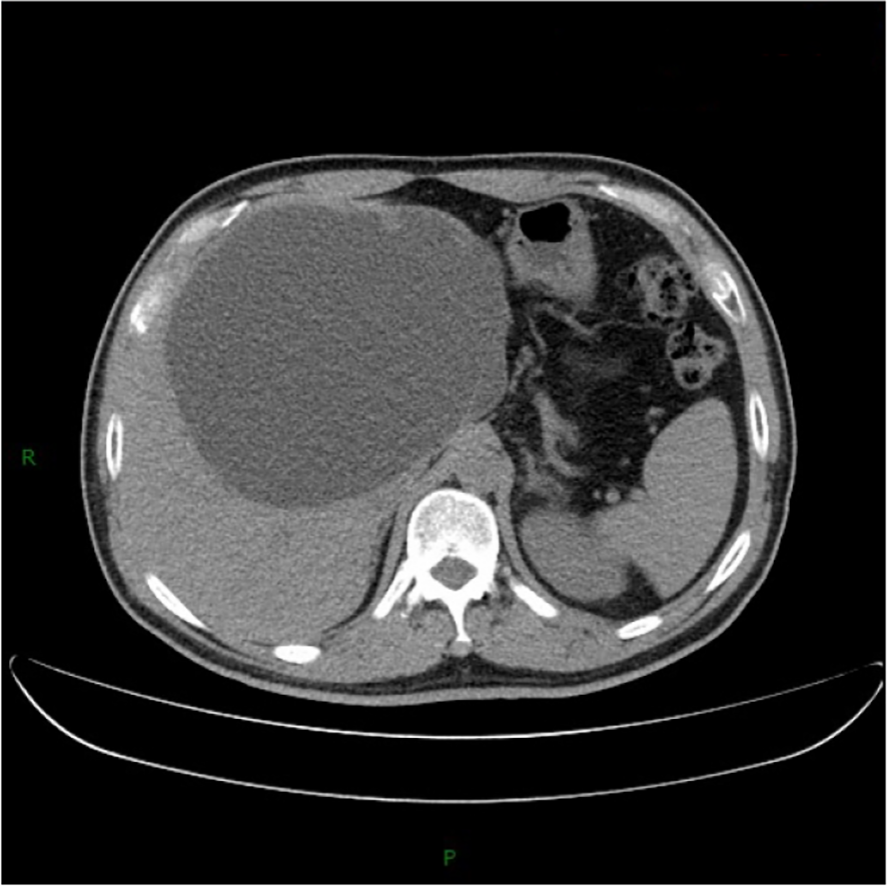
Noncontrast CT of the upper abdomen demonstrates a large cystic lesion in the left hepatic lobe measuring approximately 17 cm in greatest dimension, compatible with a giant hepatic cyst. Further characterization with contrast-enhanced CT of the upper abdomen is recommended.
